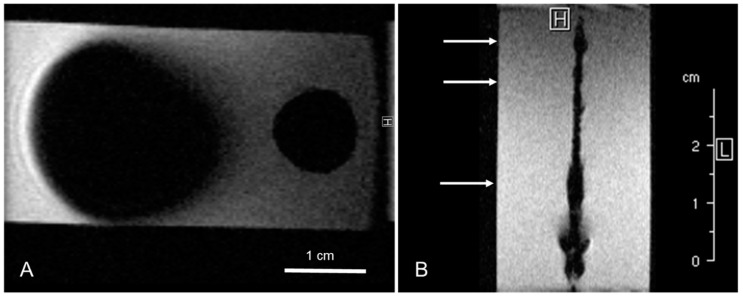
MRI image of varnish samples with a volume of 4 mm³ (A) with Bayoxide E8706 (left) and KLB particles (right). The resulting signal voids are comparably larger for Bayoxide E8706 than for KLB. (B) A guidewire with three KLB markers in different concentrations (c(Fe) = 2.4 mg/mL, 1 mg/mL and 0.5 mg/mL). In contrast to (A), the amount of KLB particles on the guidewire caused no sufficient signal voids at the real marker positions (arrows). The beading at the bottom end is probably caused by contamination of the sample. The B_0_ field direction was perpendicular to the imaging slice.
